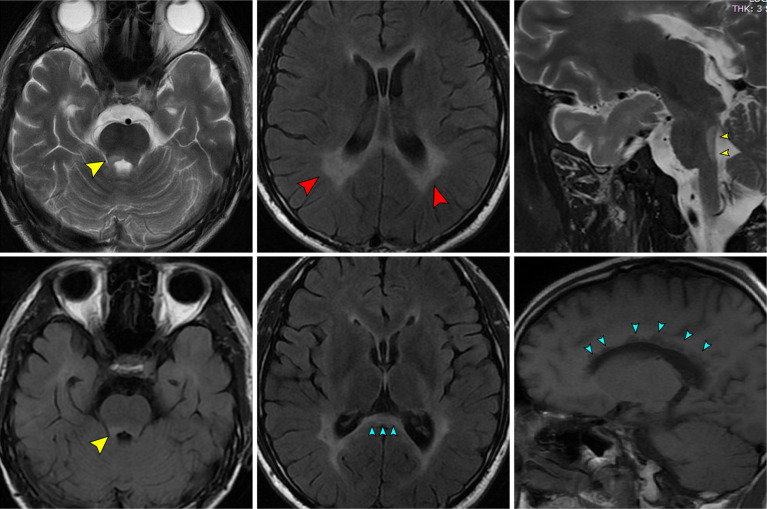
The patient’s MR images which mimicking NMOSD.
